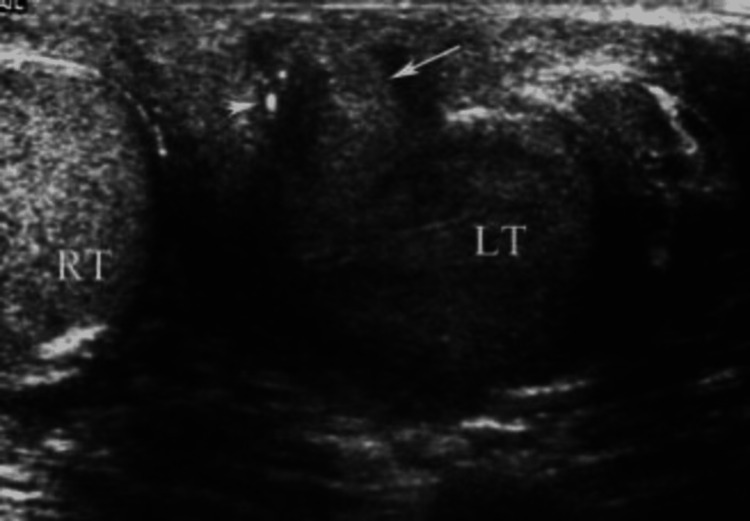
An ultrasound of the left testis shows a small sinus tract
